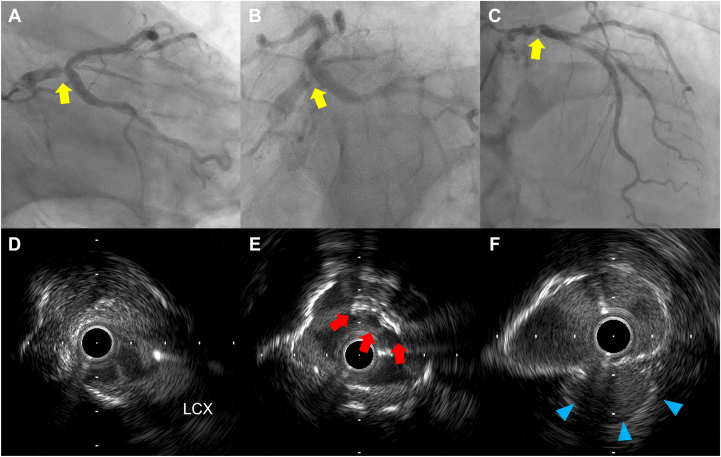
Angiographic and intravascular ultrasound findings. (A-C) Preangiographic findings. (D-F) Preintravascular ultrasound (IVUS) findings from the distal (D) to the proximal sites (F). IVUS demonstrates a calcified nodule (E; red arrows) and plaque rupture (F; blue arrows). IVUS, intravascular ultrasound; LCX, left circumflex coronary artery.
